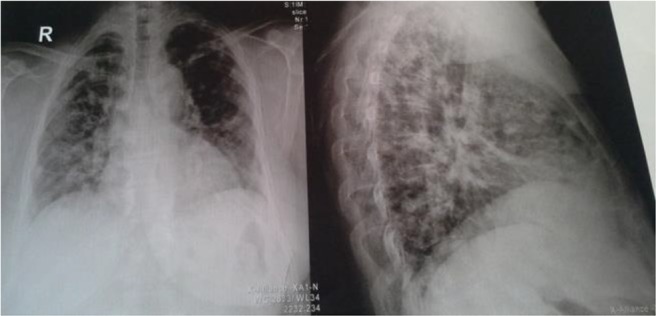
Large lobulated lesions in both the lung lobes in the chest radiography
